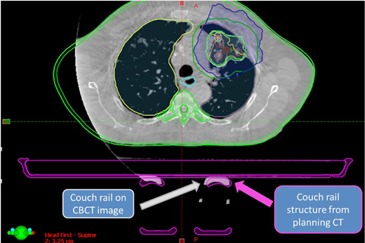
An example of treatment room verification of rail positions. The rail position can be visualized with ‘Rails In’ configuration, and that position can be compared to the couch rail contour from the treatment plan. It should be noted that the rails would be outside the CBCT FOV for ‘Rails Out’ configuration.
